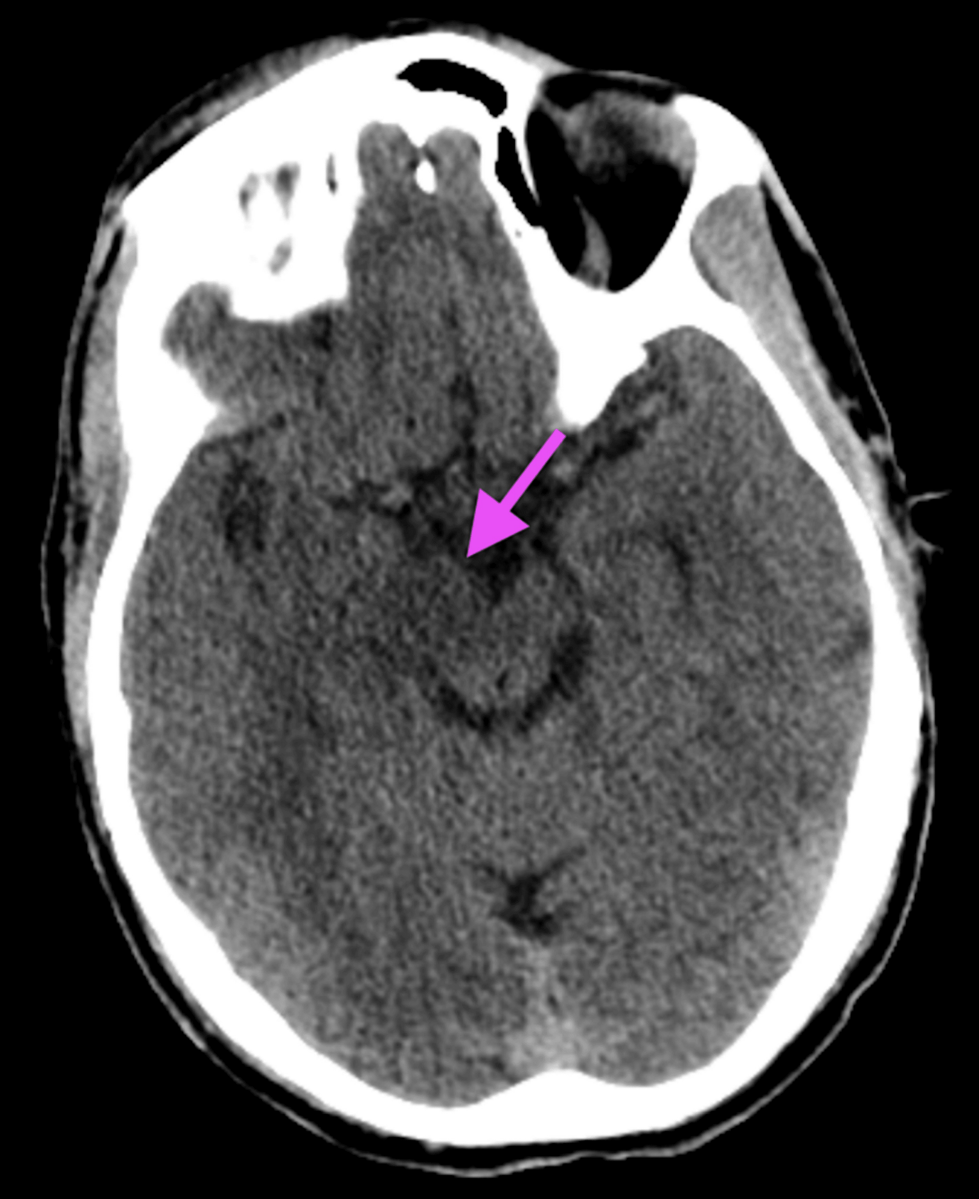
CT head A CT scan of the head shows an asymmetrical edema in the right cerebral peduncle (arrow), which extends into the upper pons.
